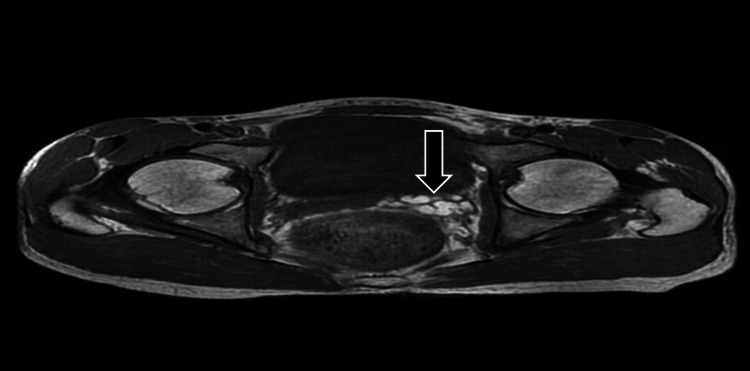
Magnetic resonance imaging (axial T1) showing hyperintense cysts on the left seminal vesicle likely to represent hemorrhagic changes (arrow)
